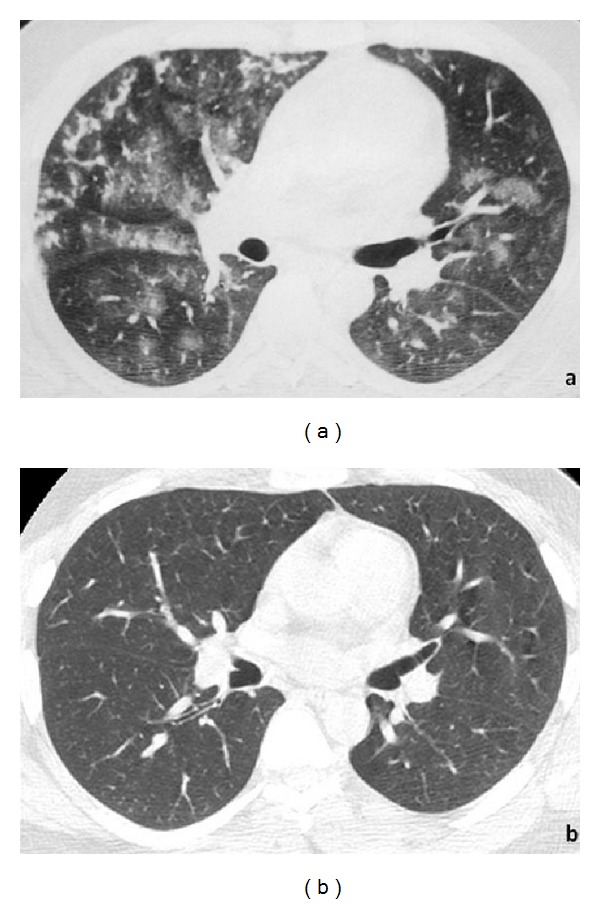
HRCT scan at the level of middle lobe at presentation (a) shows geographic and nodular areas of ground-glass opacity bilaterally as well as branching centrilobular micronodules in the middle lobe consistent with alveolar hemorrhage. HRCT scan following two month corticosteroid treatment at the same level shows complete resolution of the above mentioned findings (b).
